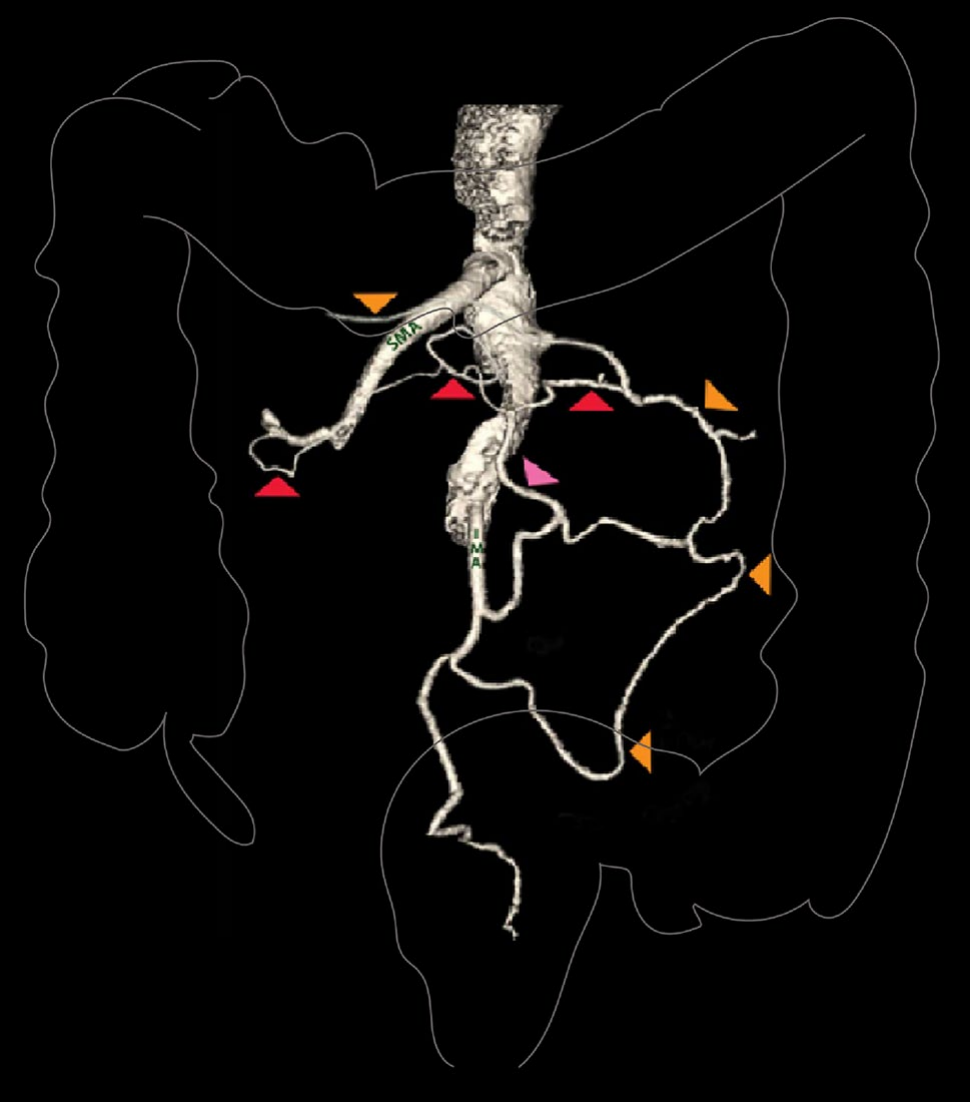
3D CT mesenteric angiogram. AoR (red arrow) joining the middle colic artery to the ascending branch of left colic artery (pink arrow). The marginal artery of Drummond is seen as a separate and distinct vessel (orange arrow)
